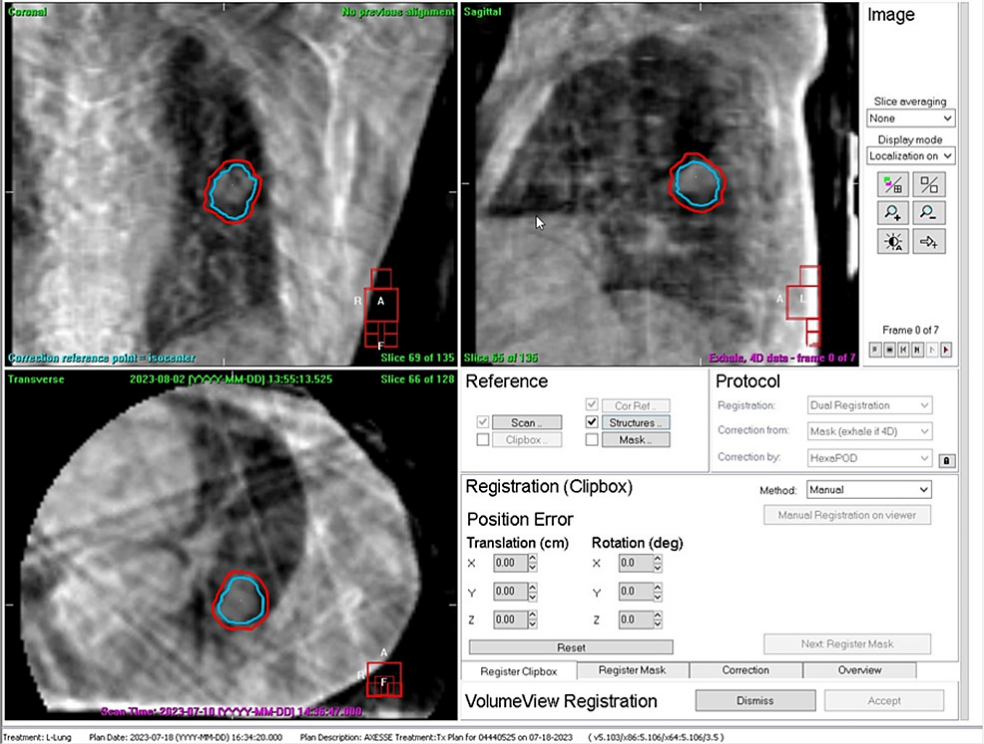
Respiratory-gated CBCT images acquired during stereotactic gated IMRT for a lung tumor case in Video 2 using a noncoplanar seven-port sliding-window technique with couch angles of 0°, 10°, and 350°. Specifically, the intrafraction half-scan CBCT images were acquired immediately after the first ports were completed with a 0° couch angle, with the linac gantry manually rotated 200° for CBCT imaging each time the measured respiratory signal amplitude met the preconfigured gate-on condition. During the CBCT data acquisition, the patient was instructed to maintain the same breathing pattern as before. In this figure, the gating window ITV is shown in sky blue and the planning target volume (PTV) is shown in red. The tumor appeared to remain within the gating window ITV during the stereotactic gated sliding-window IMRT. CBCT: cone-beam computed tomography; IMRT: intensity-modulated radiotherapy; ITV: internal target volume; PTV: planning target volume
